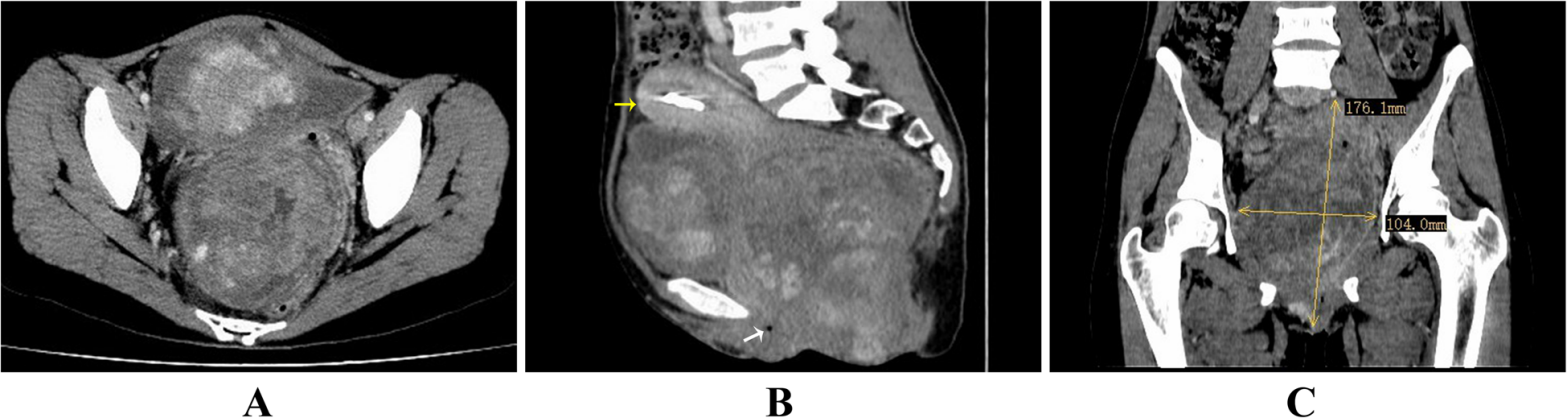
The computed tomography images of the patient with vaginal dedifferentiated liposarcoma. Abdominal computed tomography (CT) showed a 17.6 cm× 10.4 cm solid mass in pelvic cavity. Transverse (a), sagittal (b) and coronal (c) CT images revealed the tumor mass was highly suspected to originate from vagina. The yellow arrow indicate uterus, and the white arrow indicate the part of vaginal space
